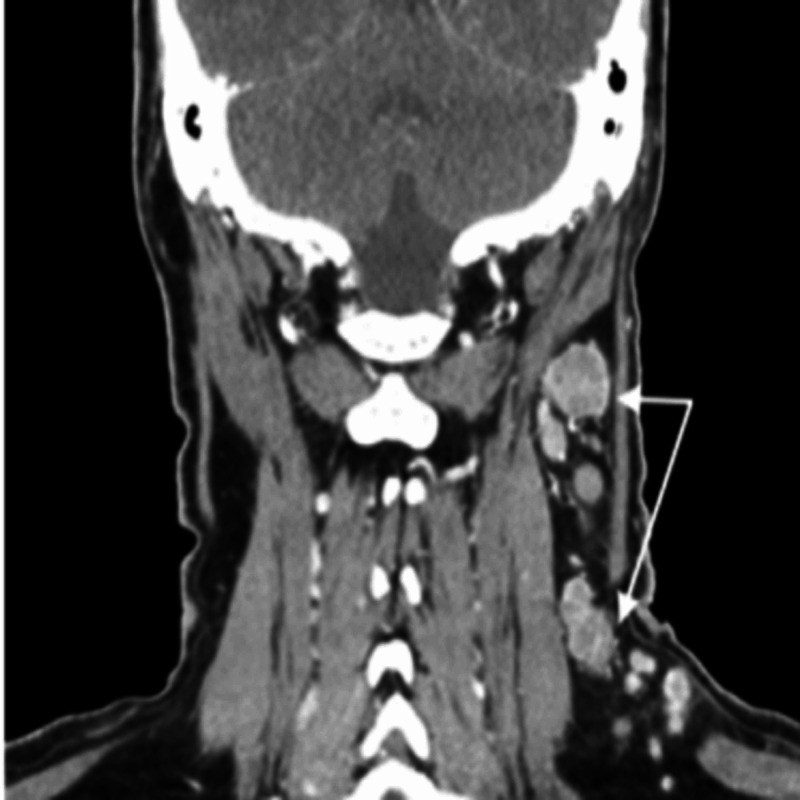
Coronal section of CECT demonstrating enlarged left upper posterior and mid jugular lymph nodes. Some of the nodes have central hypo enhancement with peripheral ring enhancement suggesting a necrotic process (marked with white arrows) CECT: contrast-enhanced computed tomography
